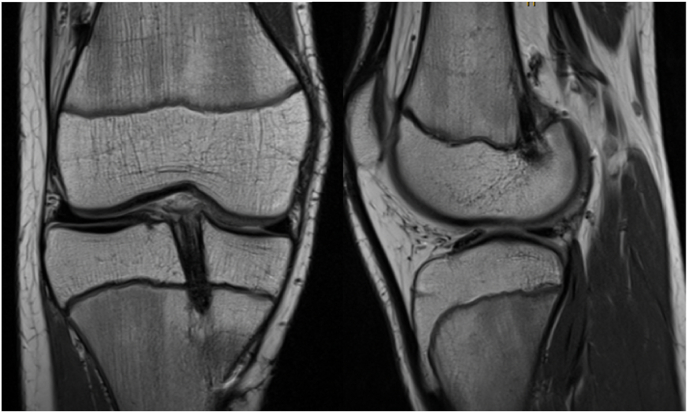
MRI scan showing no physeal bar formation adjacent to tibial or femoral graft.
